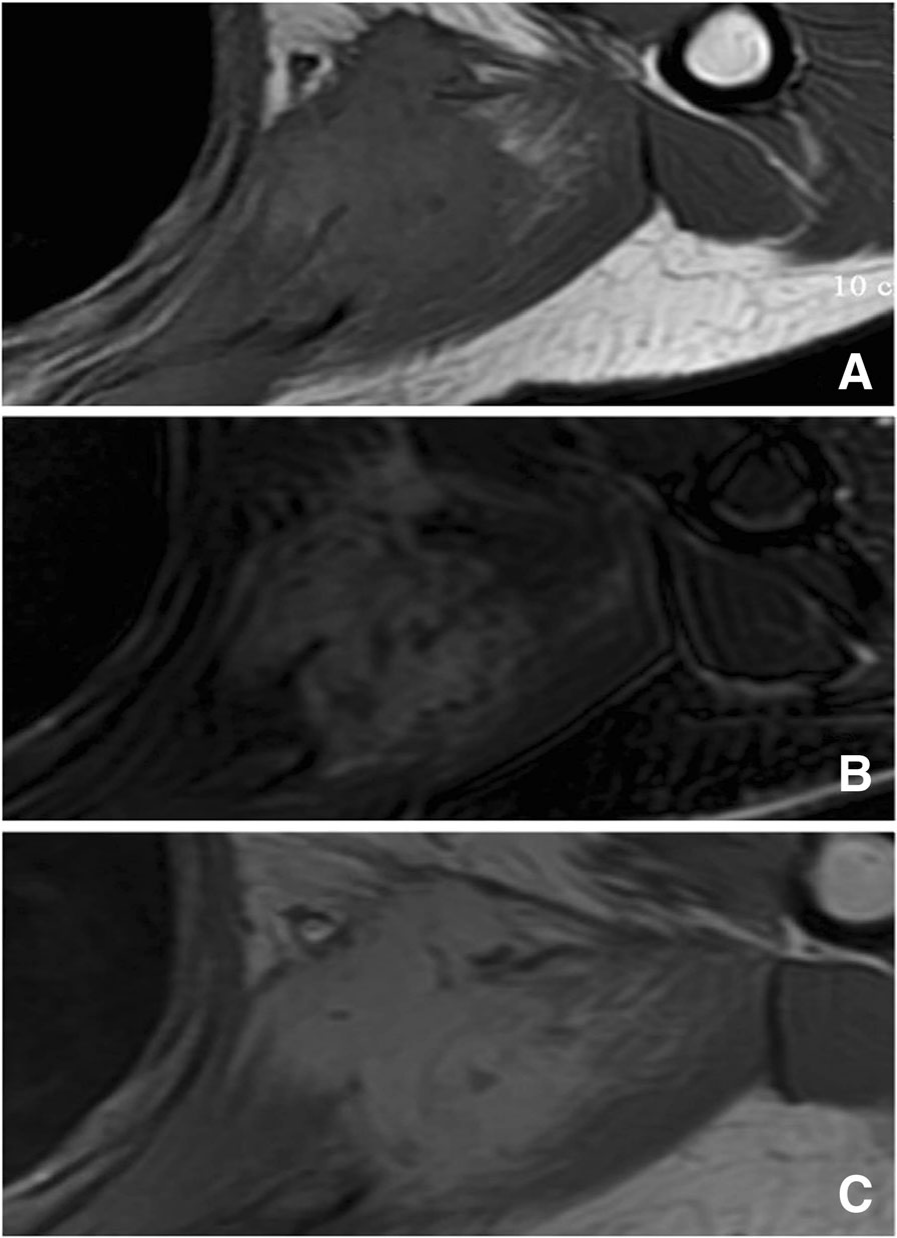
LGMS of the left lobe of the liver. CT scan revealed a low-density mass (a). The contrast-enhanced images showed heterogeneous enhancement at the early period (b). Delayed contrast-enhanced images revealed partially-filled concentric enhancement
